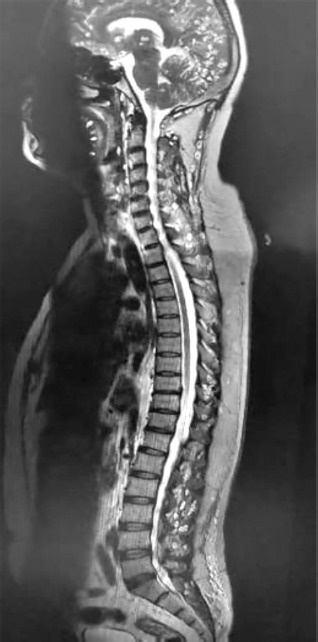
Description: Sagittal T2-weighted MRI of the cervicothoracic and lumbar spine demonstrates reversal of cervical lordosis with diffuse disc bulges at multiple cervical levels causing anterior thecal sac indentation. The spinal cord appears normal in caliber and signal intensity, without evidence of focal myelomalacia. The lumbar spine reveals disc desiccation with diffuse disc bulge at the L4–L5 level, producing anterior thecal sac indentation. No abnormal intramedullary signal changes or compressive myelopathy are observed. Clinical Significance: These findings are consistent with degenerative disc disease involving both the cervical and lumbar spine. Recognition of disc pathology at multiple levels is crucial for guiding conservative versus interventional management and for ruling out early compressive myelopathy. Origin: @GSVM Medical College, PMSSY Superspeciality Hospital, Kanpur, Uttar Pradesh, India. Pincode: 208002.
